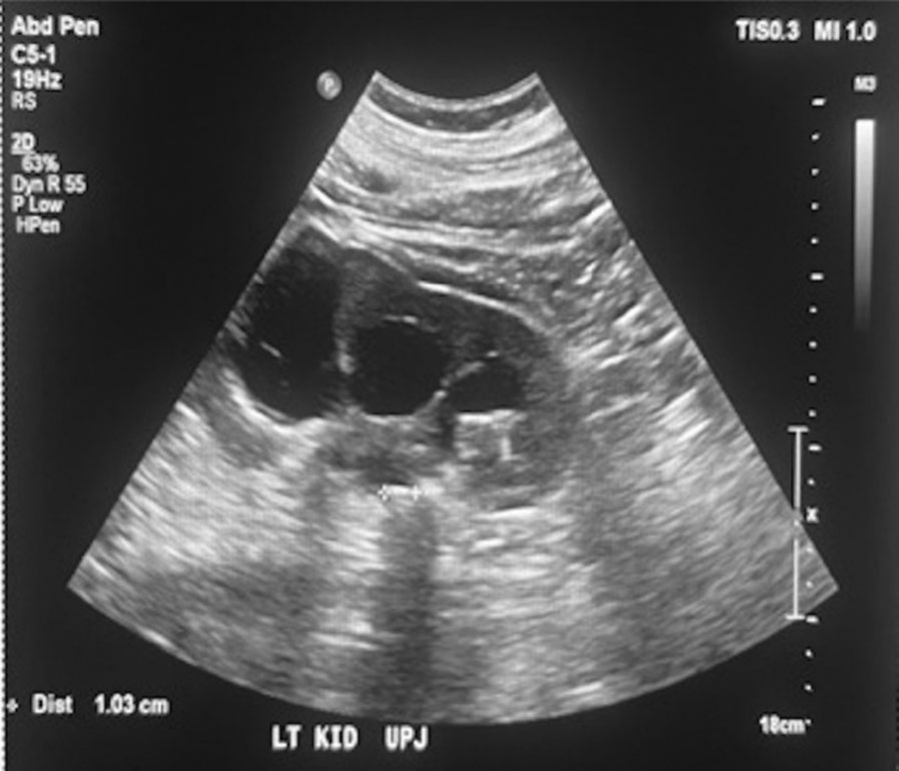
Renal ultrasound showing an obstructive 1-cm calculus at the left UPJ, causing moderate to severe hydronephrosis
